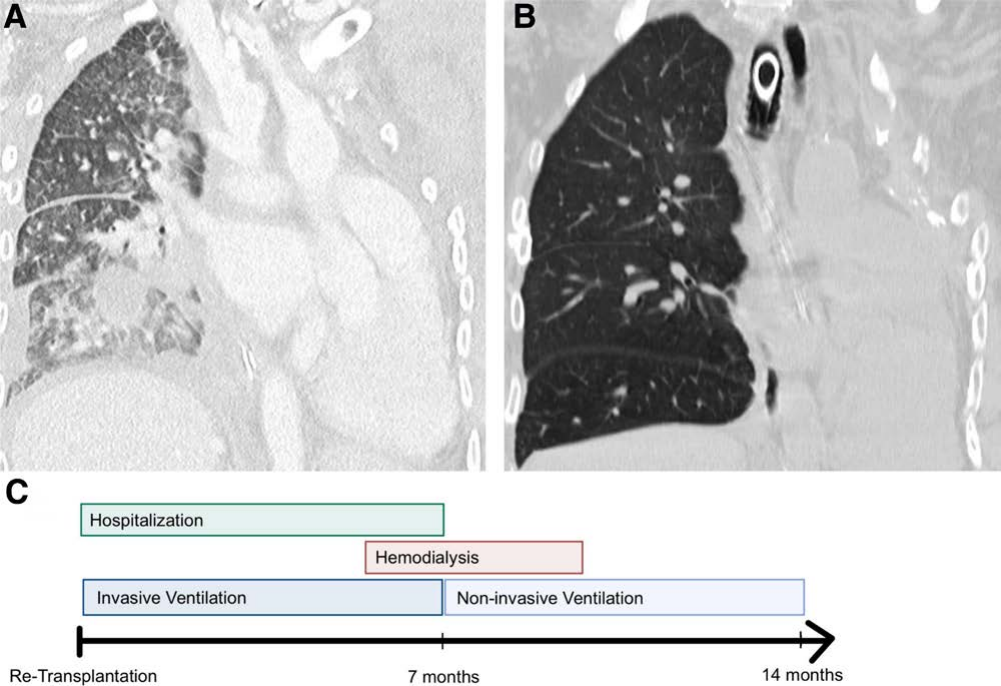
Computed tomography revealed interstitial and alveolar edema at 1 mo postoperatively (A). All infiltrates had completely resolved when the patient was discharged for rehabilitation several months later (B). The postoperative clinical course was prolonged because of the necessity of hemodialysis and invasive ventilation (C).
